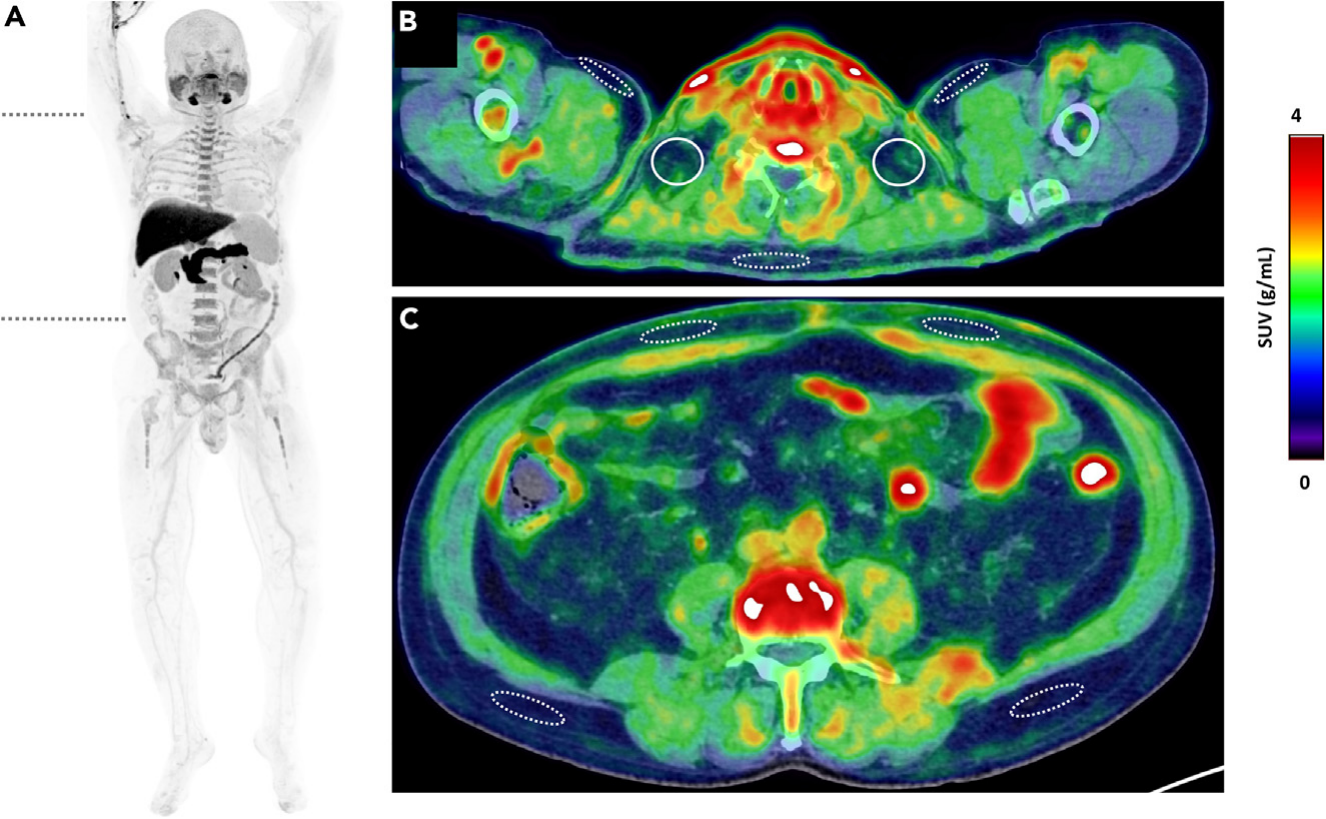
Normal ^18^F-fluciclovine biodistribution Maximum intensity projection image of a 61-year-old man (183 cm, 98 kg) with biochemical recurrence of prostate cancer, demonstrating physiologic ^18^F-fluciclovine uptake, being most prominent in the pancreas, liver, salivary glands, and bone marrow. The two transverse dashed lines on (A) point to the level of cross-sectional axial fused PET/CT images (B) and (C), demonstrating supraclavicular adipose tissue depot (solid circles, B), upper chest subcutaneous adipose tissue (dashed ellipses, B), and abdominal subcutaneous adipose tissue depots at various locations opposite third lumbar vertebra (dashed ellipses, C). Visually, the supraclavicular regions show subtle higher ^18^F-fluciclovine uptake compared to other regions shown in (B) and (C). The difference was more evident on quantification.
